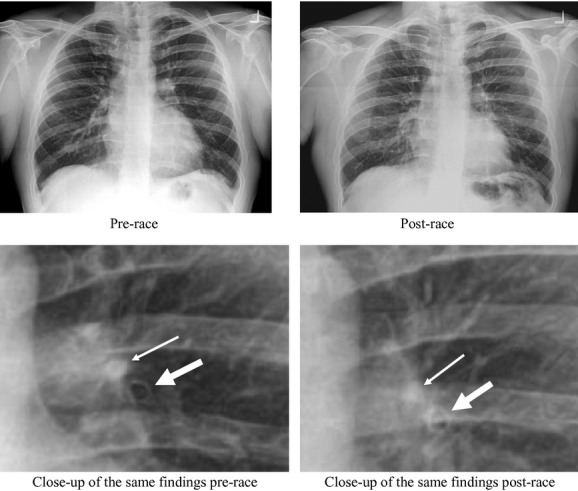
Evidence of postmarathon increase in peribronchial (thicker arrows, bottom) and perivascular (thinner arrows, top) cuffing. Subject was male, 32 years old, BMI = 27.7 kg/min^2^. He completed the marathon in 4 h 25 min. The postexercise radiograph was obtained 20 min post marathon finish.
